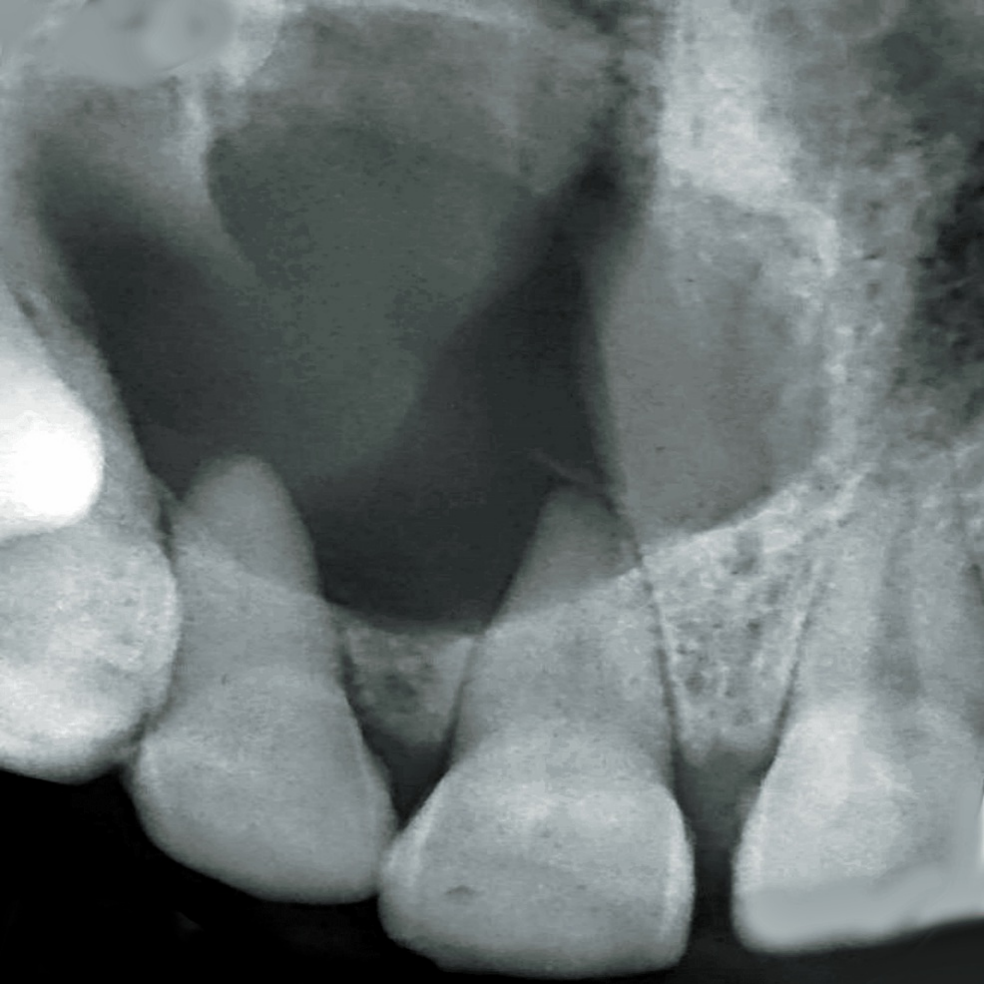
Intraoral periapical radiograph with 11-13 region. Image Credit: Prasanna Sonar.
